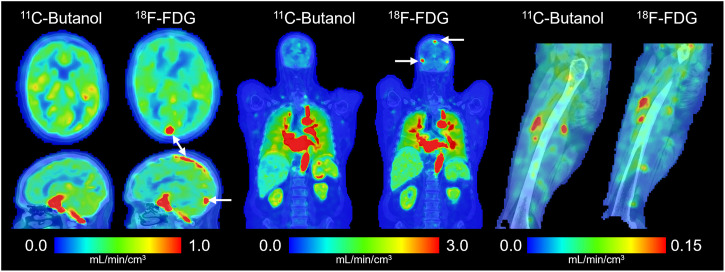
Total-body parametric imaging of blood flow with early dynamic ^18^F-FDG method compared with ^11^C-butanol flow-tracer PET reference in same participant. White arrows indicate sagittal and transverse sinuses in brain.
